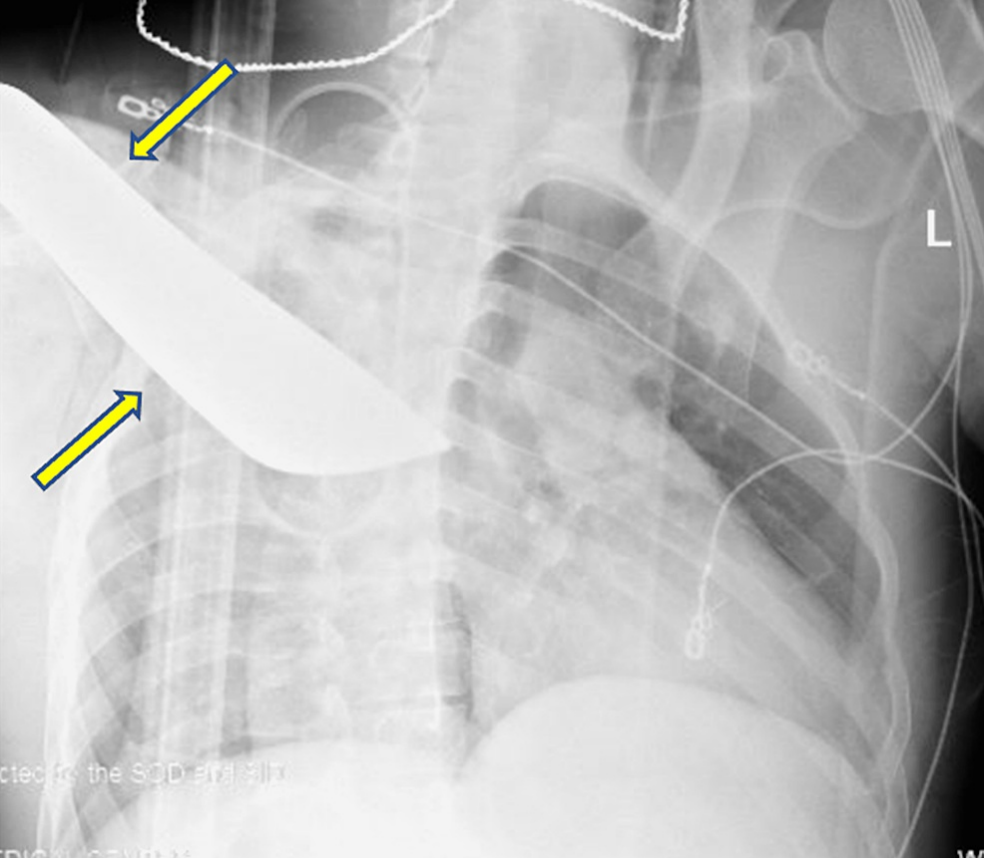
Chest X-ray taken in the trauma bay prior to surgical intervention shows the impalement object (arrows), and no obvious hemothorax, pneumothorax, or identifiable lung injury.
